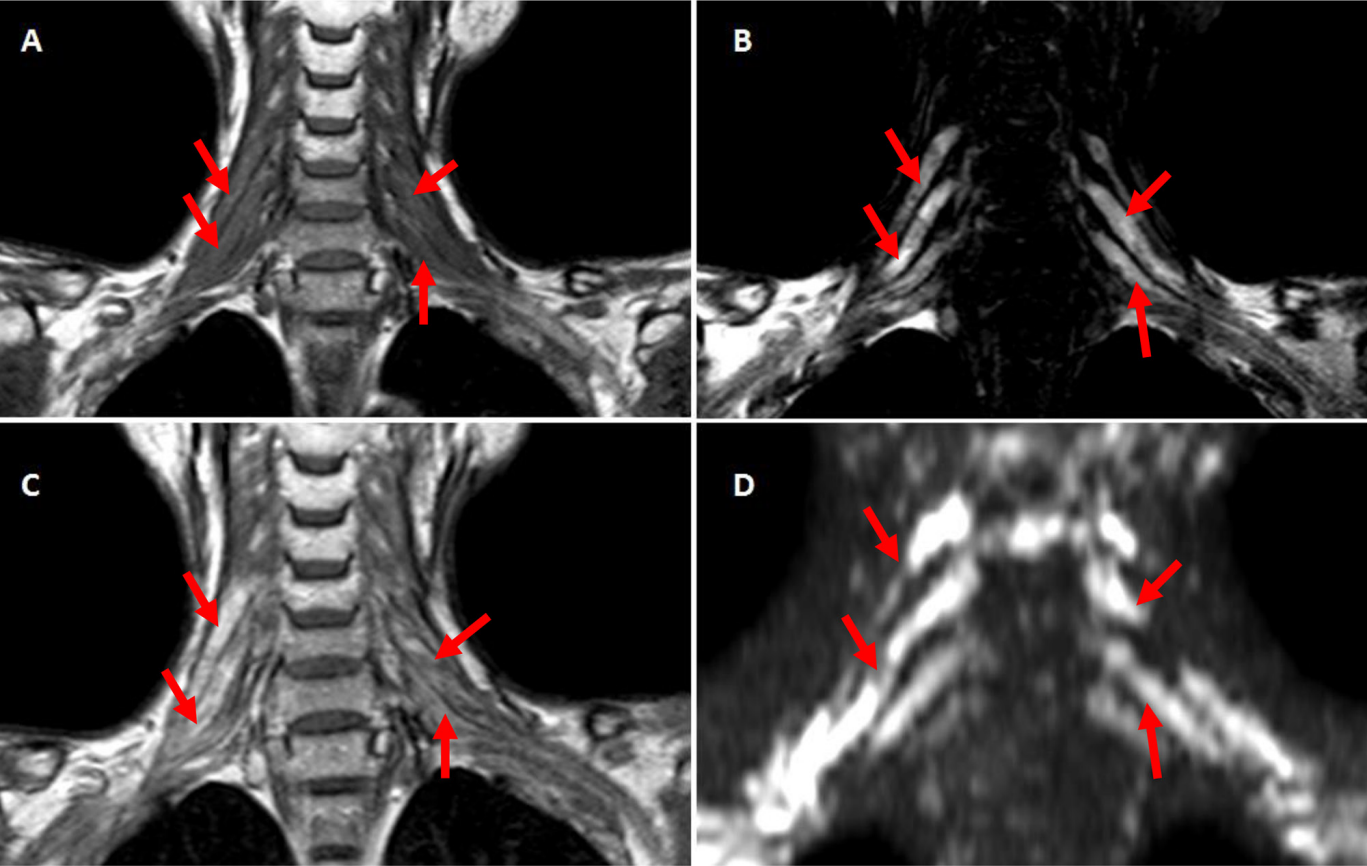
A-D. The coronal MRI scan of one case with bilateral RIBP T1-weighted coronal MRI scan showed bilateral brachial plexus were swollen with hyper-intensity. (A) C-5∼C-8 roots and corresponding trunks of brachial plexus showed prolonged T2 relaxation time at T2-weighted MR imaging (B), high intensity at Post-contrast T1-weighted imaging (C) and DWIBS (D) (indicated with red arrows).
